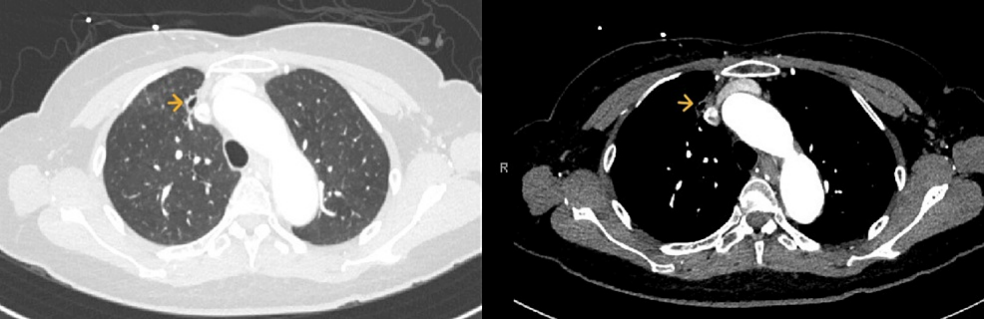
Chest CT Chest CT scan with contrast showed a small cavitary lesion in the right upper lobe and subtle bilateral lower lobe ground glass opacities.
